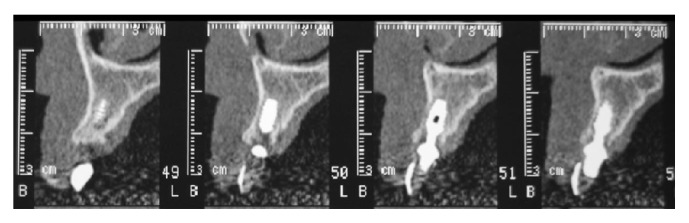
CT scans three weeks after surgery. Note the absence of vestibular bone dehiscence.
